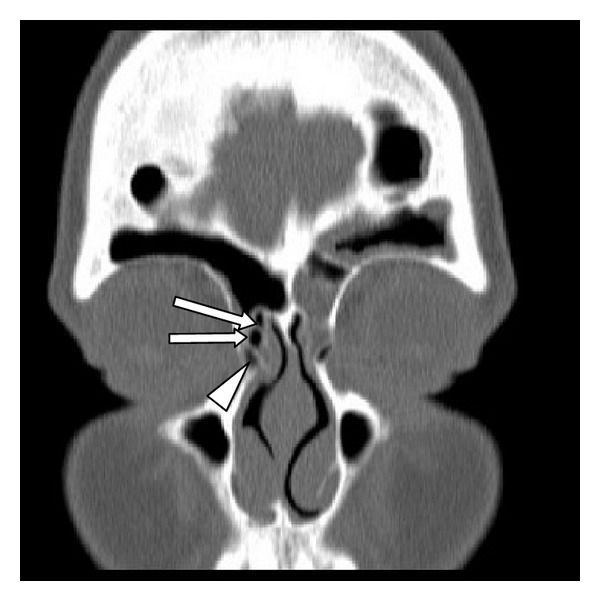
Type II frontal cells and agger nasi cell. A coronal CT scan showing right-sided type II frontal cells (arrows) above an agger nasi cell (arrow head).
